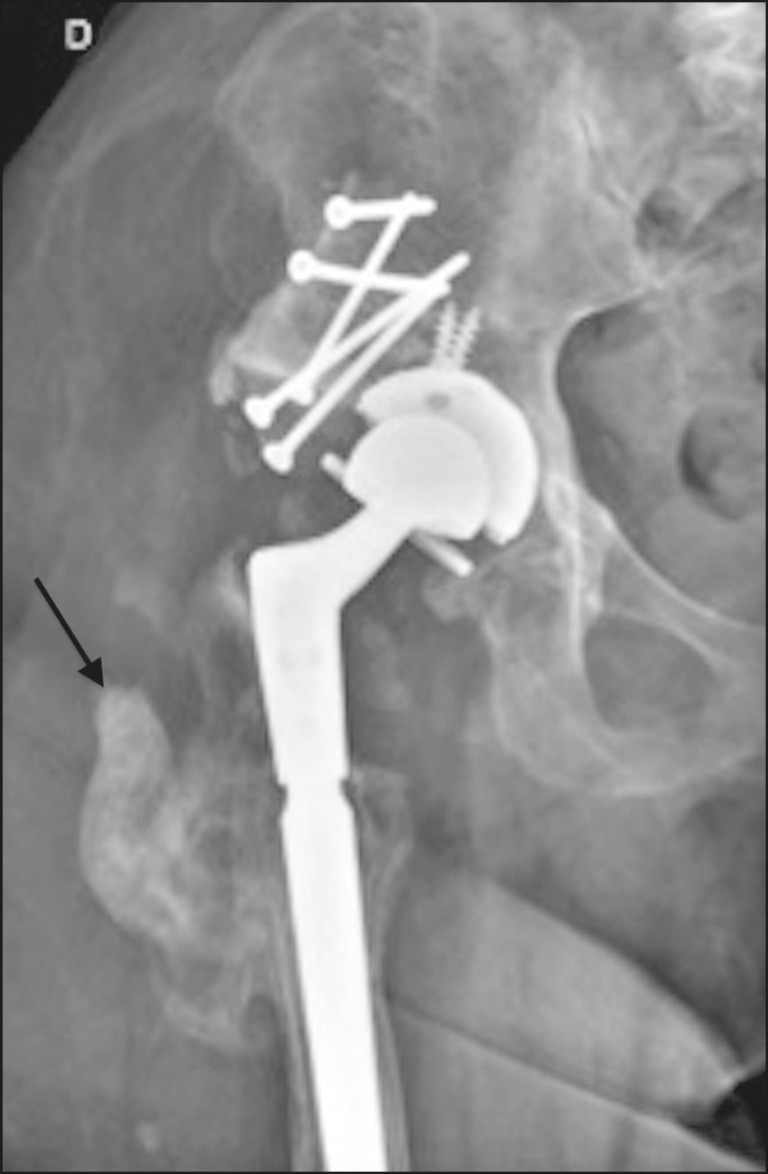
Anteroposterior X-ray of the right hip showing signs of total arthroplasty and bone grafting, with no signs of loosening. Grade III heterotopic ossification of soft tissue near the sites of surgical manipulation (arrow).
